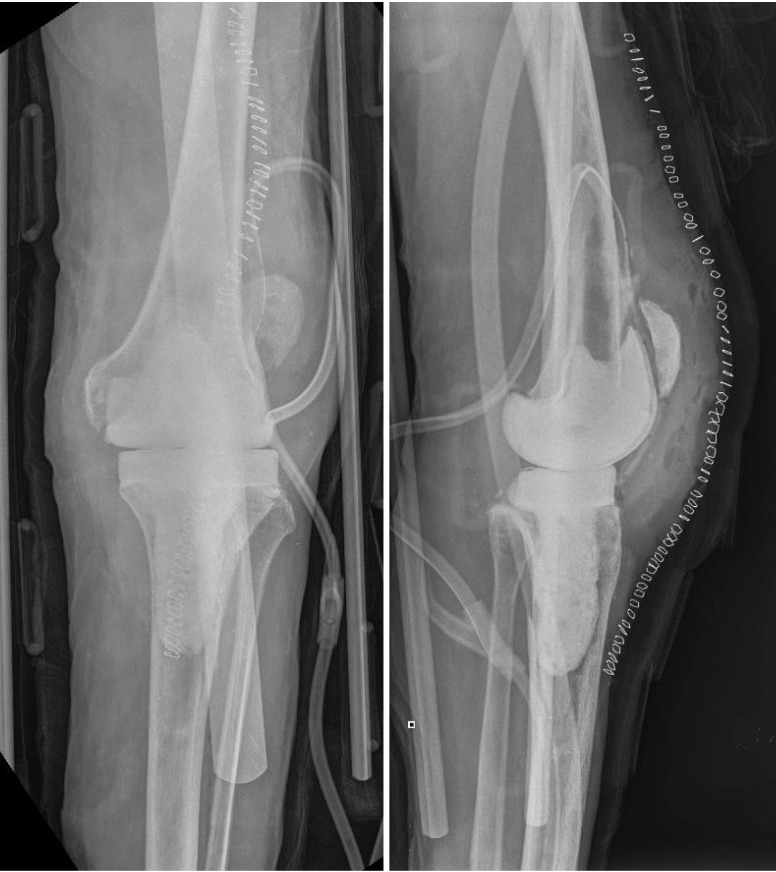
9/30/2013. Postoperative AP (A) and Lateral (B) radiographs demonstrating appropriate alignment of the knee after explantation of components and PMMA antibiotic spacer insertion.
